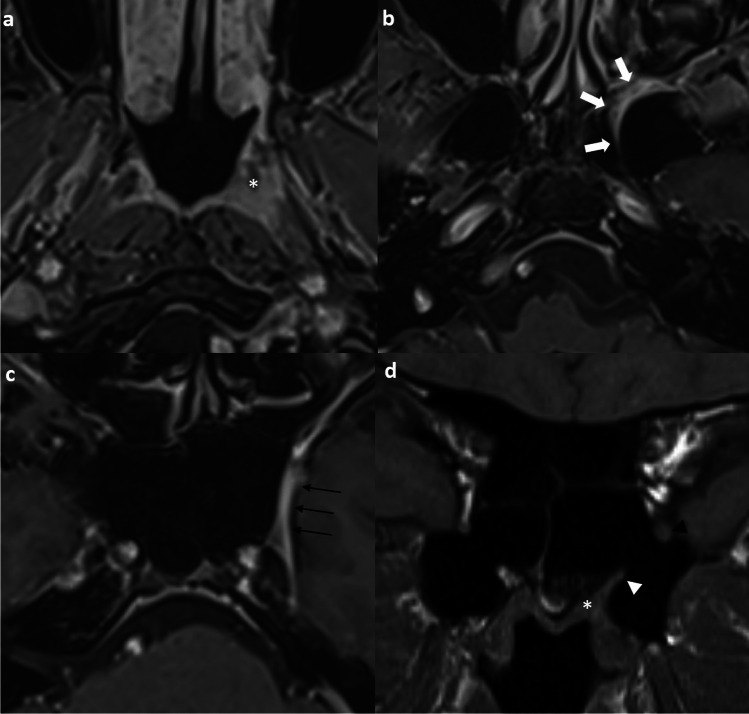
Perineural tumor spread in a 60-year-old man presented with left maxillary facial numbness with biopsy-proven undifferentiated carcinoma in left nasopharynx. Axial post-contrast T1-weighted image with fat suppression shows the left nasopharyngeal tumor at the fossa of Rosenmüller (asterisk) (a). Axial post-contrast T1-weighted image with fat suppression at a higher level reveals asymmetric enlargement and enhancement of the left vidian canal extending anterolaterally into the left pterygopalatine fossa (arrows) (b). Axial post-contrast T1-weighted MR image with fat suppression at a further higher level shows asymmetric enlargement and enhancement of the left foramen rotundum along the cavernous sinus (arrows) (c). Coronal T1-weighted image without fat suppression shows the asymmetric enlargement of the left foramen rotundum (black arrowhead) and vidian canal with fat effacement (white arrowhead). The nasopharyngeal tumor extends to the left vidian canal with perineural spread (asterisk) (d). The apparent small tumor in the nasopharynx with underdetection of perineural tumor spread may lead to understaging and subsequent undertreatment with missing irradiation target and over-estimation of survival
